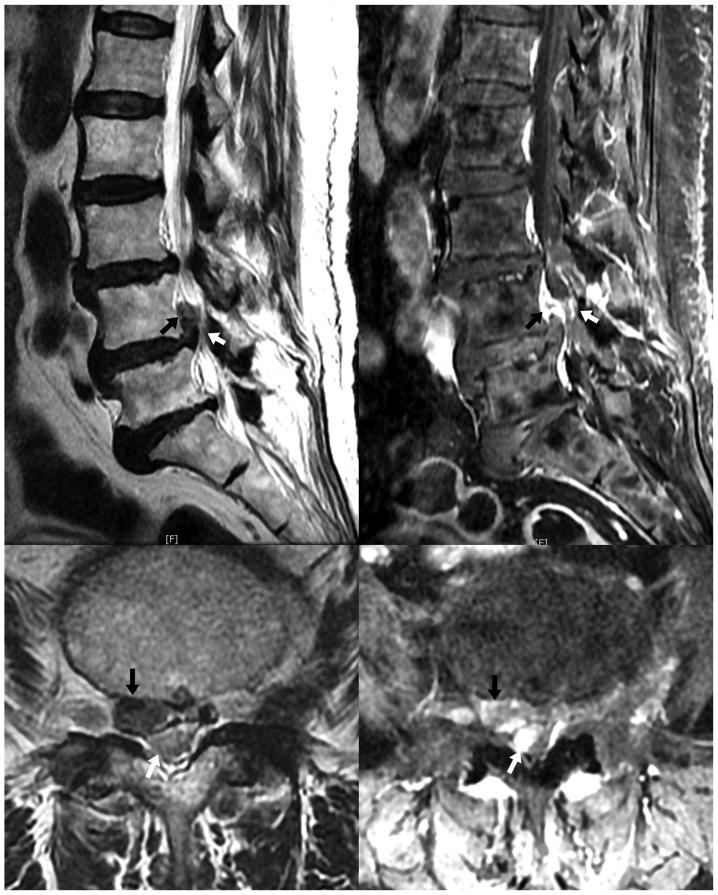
T2-weighted (left) and enhanced T1-weighted (right) sagittal (top) and axial (bottom) magnetic resonance imaging revealing disc herniation compressing the thecal sac and right neural foramen at L4/5, shown by the black arrows, and an intradural structure of increased density following enhancement at the same site, shown by the white arrows.
